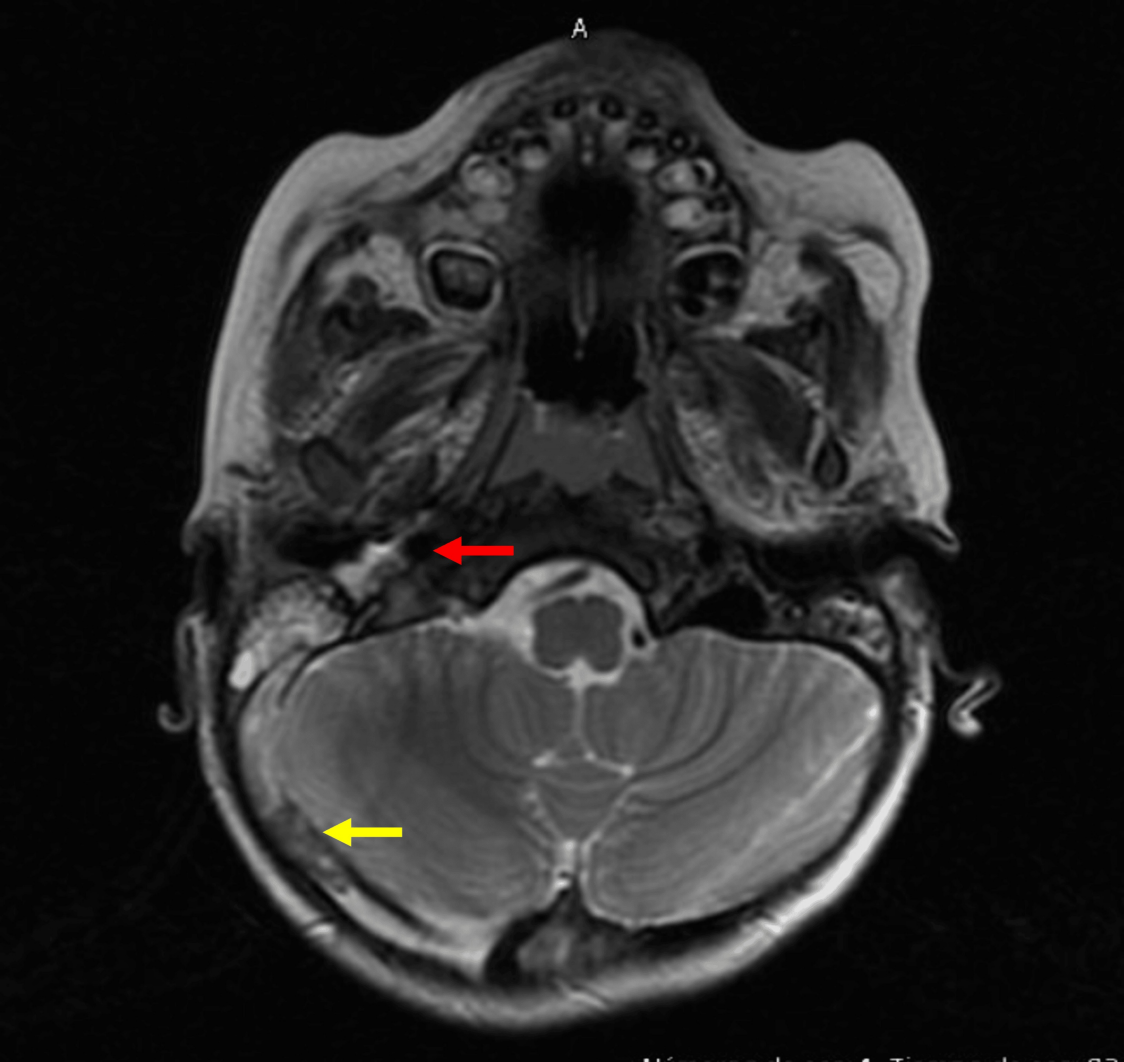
Magnetic resonance imaging: the red arrow indicates enhancement of the right jugular vein wall, suggesting thrombophlebitis; the yellow arrow shows thrombosis in the right transverse and sigmoid sinuses.
